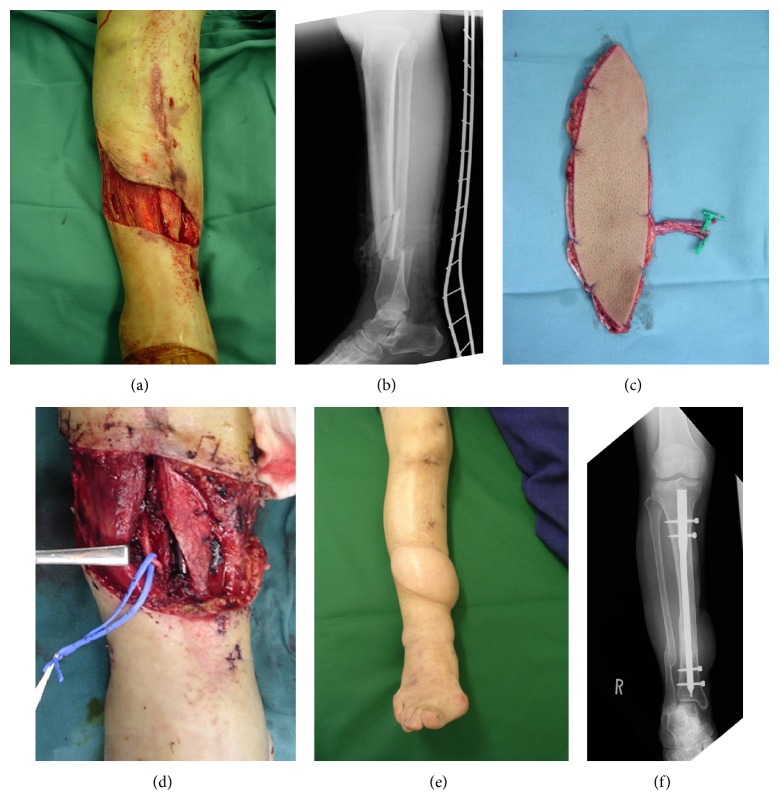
(a) Open fracture of the lower extremity is accompanied by an moderate soft tissue defect on the anterior lower extremity. (b) X-ray findings. (c) Anterolateral thigh flap harvested from the same side. (d) The anterior tibial artery was selected for end-to-side anastomosis. (e) Appearance at 7 months postoperatively. (f) X-ray findings at 7 months postoperatively, showing bone union.
